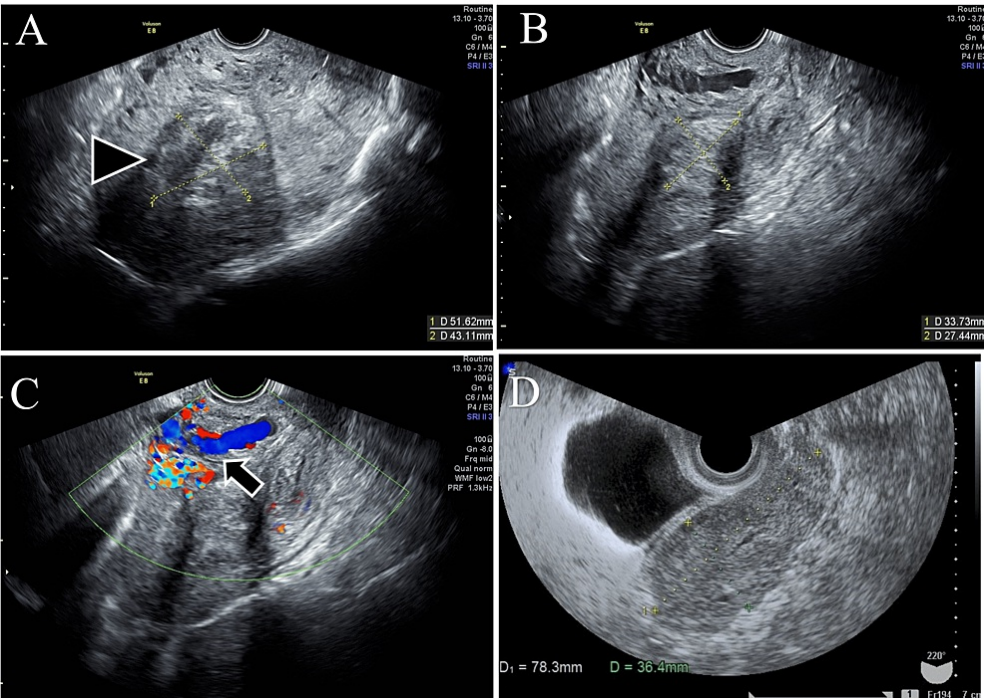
Transvaginal ultrasonography image A) Transvaginal scan on day 1 postpartum showing intrauterine mass (head arrow). B) Transvaginal ultrasonography image on postpartum day 35 showing an echogenic area in the muscular layer of the anterior wall of the uterus. C) Transvaginal color Doppler ultrasonography confirmed angiogenesis, with abundant blood flow in the same area (arrow). D) Transvaginal ultrasound image obtained 78 days after transcervial resection: there were no obvious pooling in the uterine cavity, and the angiogenesis shown in Figure C had disappeared.
